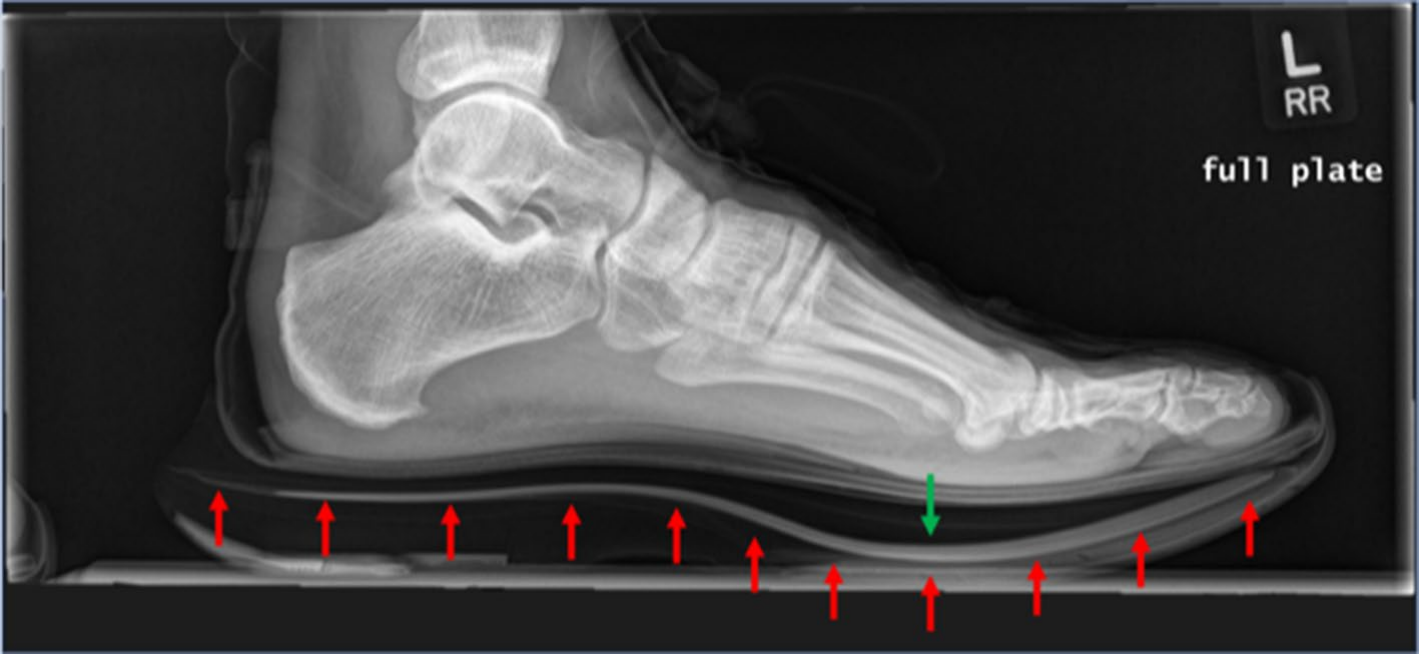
Lateral X-ray of a runner's left foot in a carbon-plated running shoe. Red arrows outline the embedded plate. The green arrow shows the fulcrum point of the plate. Note the relation of the curvature of the plate to the metatarsal locations (metatarsal phalangeal joints)
